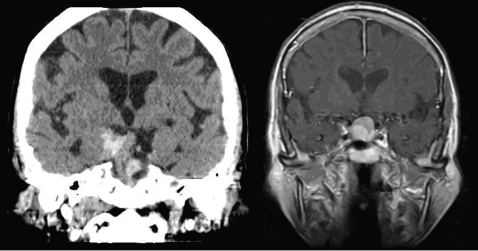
Coronal views of head CT (left) and MRI T1 with contrast (right) showing hemorrhage in the pituitary macroadenoma.
